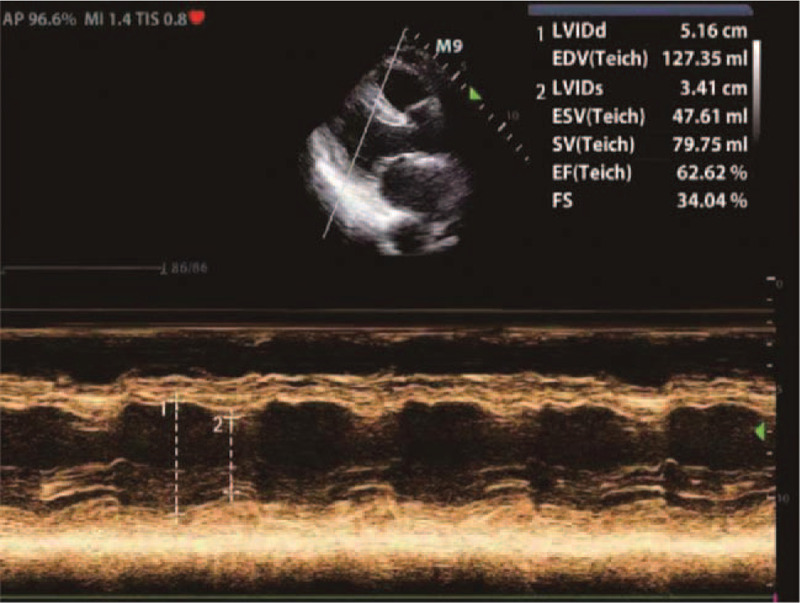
Echocardiography showing a left ventricular ejection fraction (LVEF) of 62.62%.
